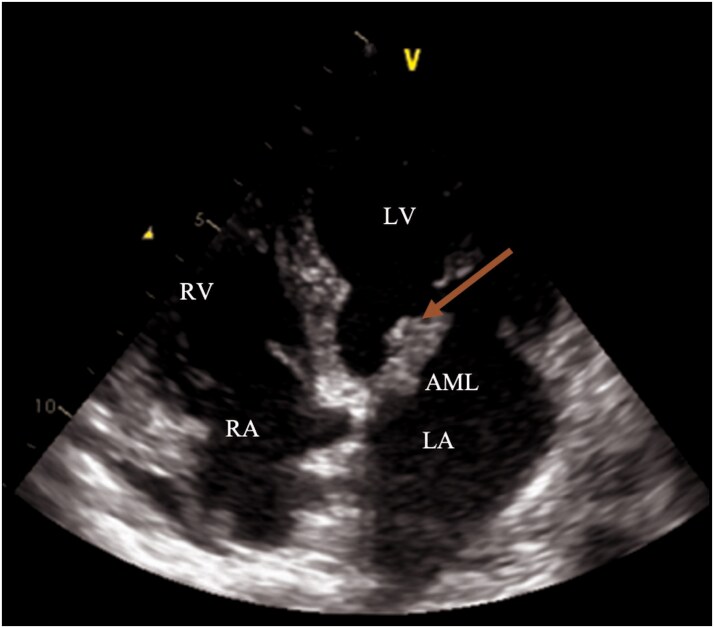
Transthoracic echocardiography (13 April 2023), four-chamber view. A mass extending on the anterior mitral leaflet is visualized, measuring 18× 7 mm (arrow). Anatomical landmarks: AML, anterior mitral leaflet; LA, left atrium; LV, left ventricle; RV, right ventricle; RA, right atrium.
